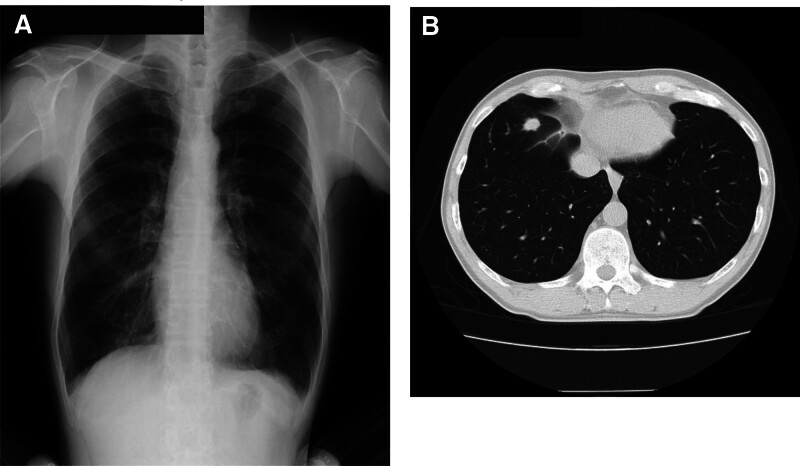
CR and LDCT images of lung cancer case taken on the same day. (A) CR reveals no abnormal opacity. (B) LDCT shows a tumorous lesion in the right lower lung field. This patient was diagnosed with stage I lung cancer. CR = chest radiography, LDCT = low-dose computed tomography.
